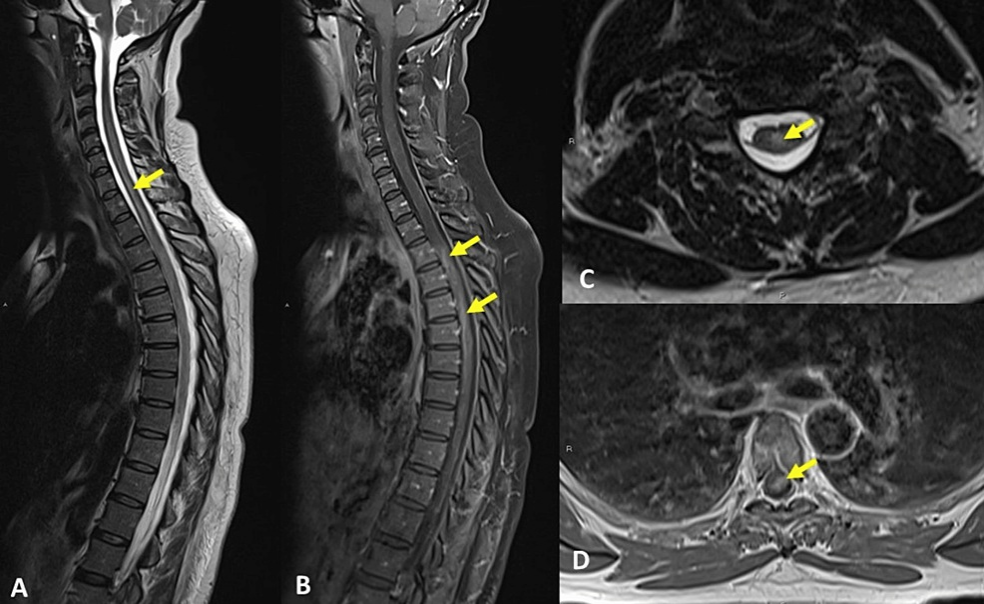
MRI of the spinal cord - (A) sagittal T2-weighted sequence, (B) sagittal contrast-enhanced T1-weighted, (C) axial T2-weighted (C6 level), and (D) axial contrast-enhanced T1-weighted (T5 level). Neuroimages reveal central spinal cord swelling from C4 to T10 and increased T2-weighted signal in C6, C7, and T1 levels (A, C - arrows). Following gadolinium administration, a patchy enhancement of the spinal cord from T2 to T6 was documented, mainly on the left side (B, D - arrows). Pachymeningeal contrast enhancement was also observed (B).
